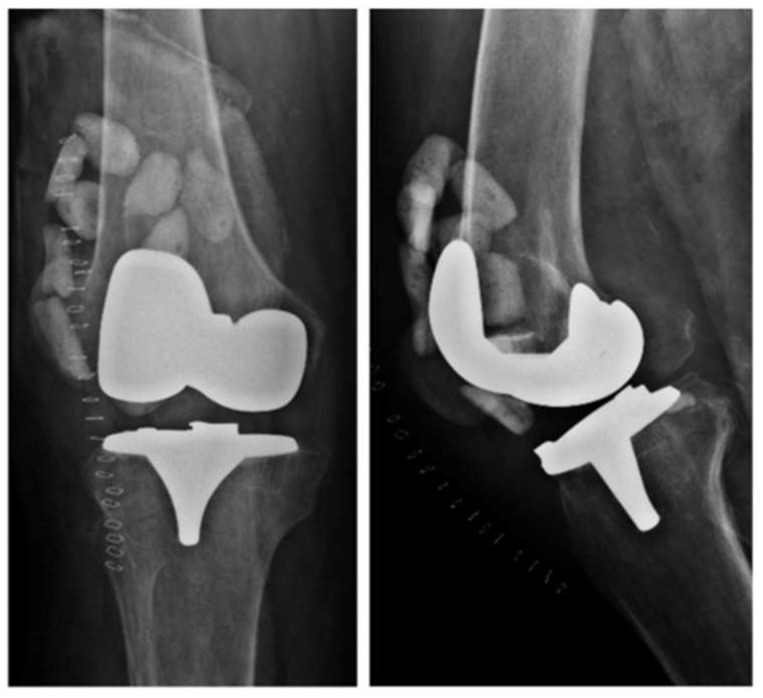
X-rays of a right knee with an infected total knee arthroplasty showing cement beads inside the joint capsule.
